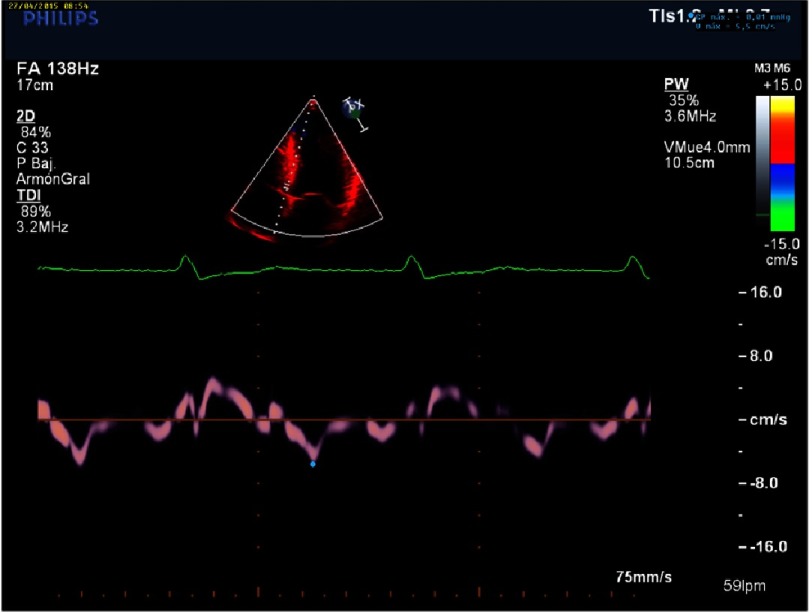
Low velocities in tissue Doppler imaging of the mitral annulus (under 8 cm/s) can be seen in early stages.
